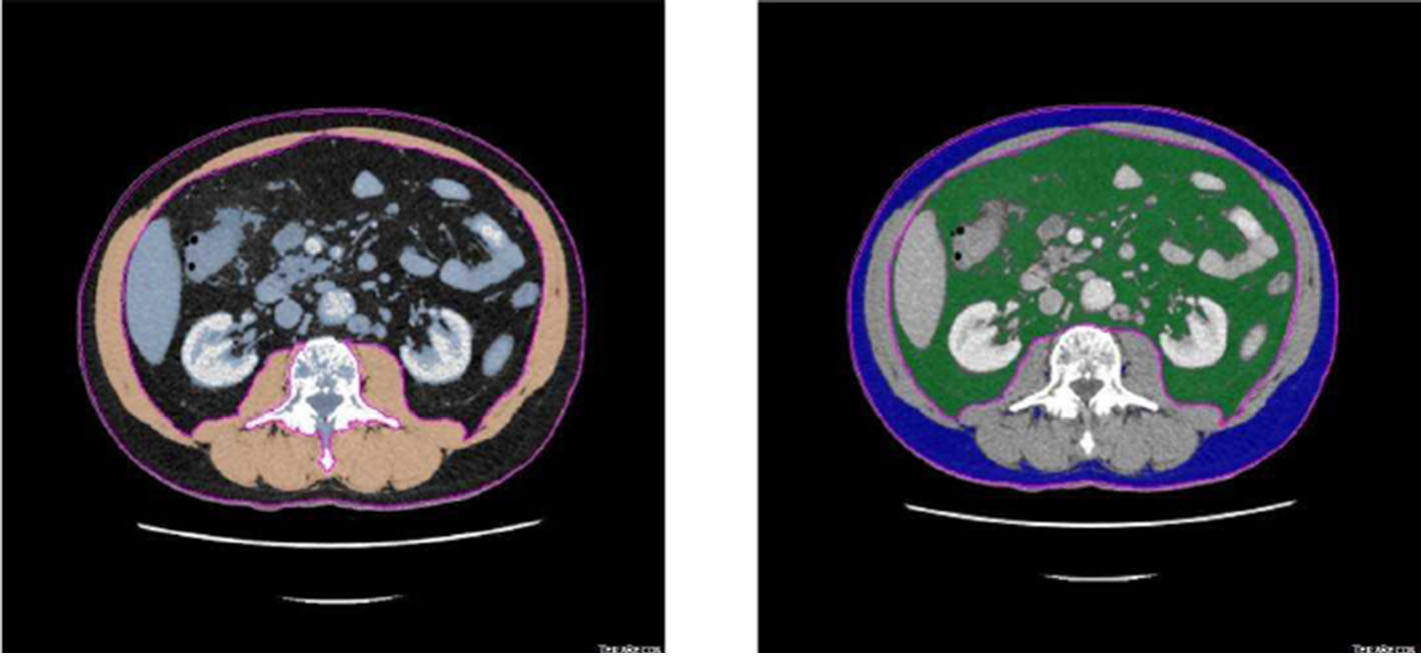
Cross sectional CT imaging at 3rd lumbar vertebra. Muscle tissue is shown in orange color (left), subcutaneous tissue in blue color, and visceral adipose tissue in green color (right)
